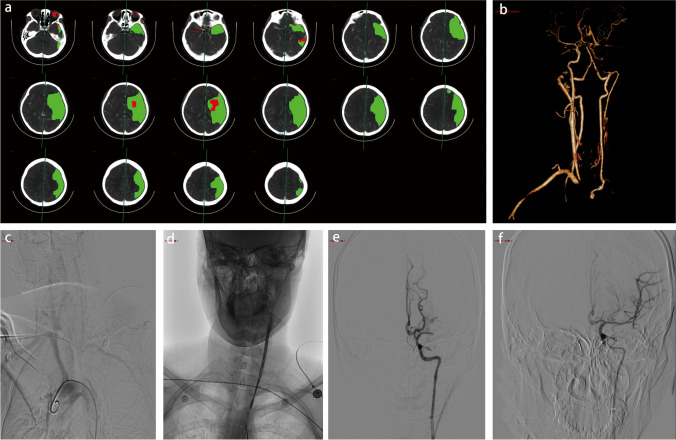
The CTP (a), CTA (b), and DSA (c–f) images. a The CTP images showed a mass of salvageable brain tissue in the left MCA territory. b The reconstructed CTA images showed occlusion of the left SCA, left ICA and M1 segment of the left MCA, and stenosis of the left CCA. c Angiography of the aortic arch showed occlusion of the proximal left SCA. d Angiography of the left CA showed stenosis of the left CCA and occlusion of the left ICA. e Angiography failed to showed the left MCA from the M1 segment. f Full reperfusion of the MCA was achieved after mechanical thrombectomy
